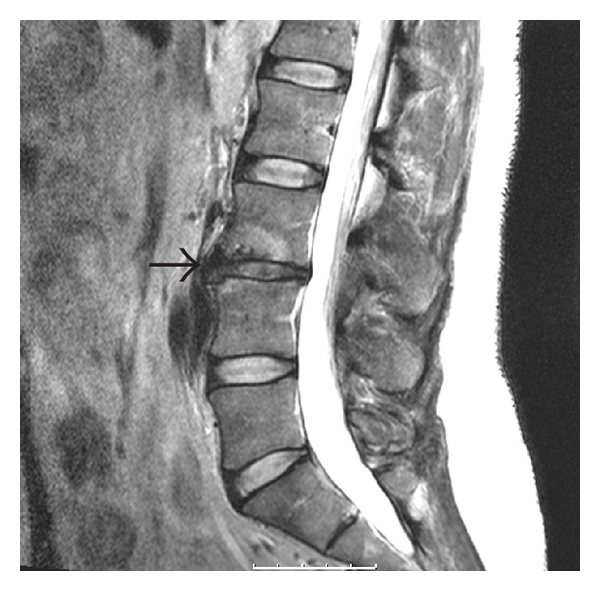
Disc space narrowing and degenerative changes at the L3-L4 level (arrow) on sagittal T2-weighted MRI.
